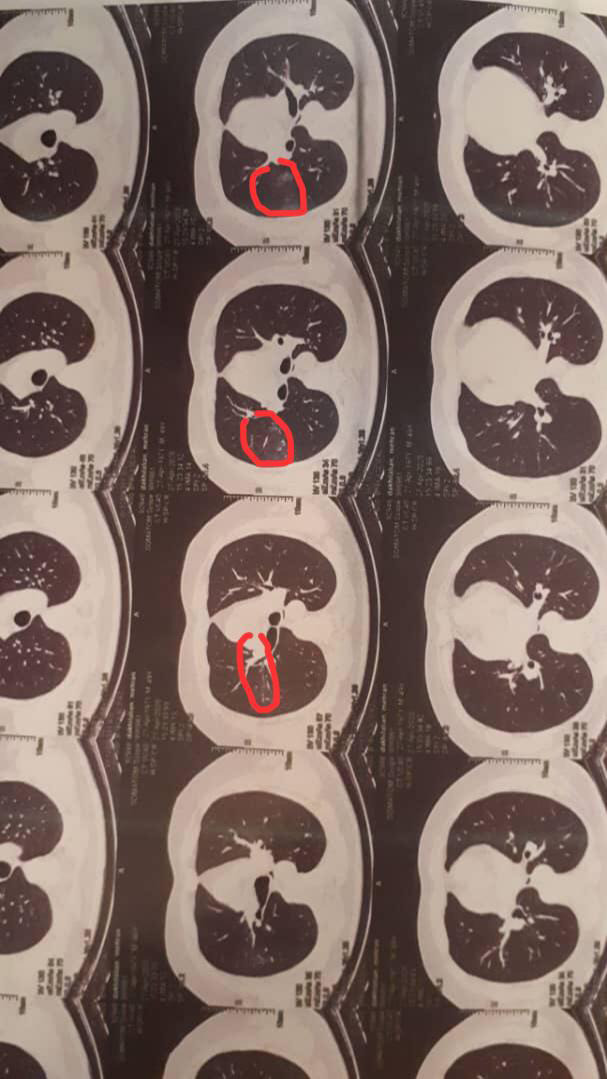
Ground glass opacity in CT scan
